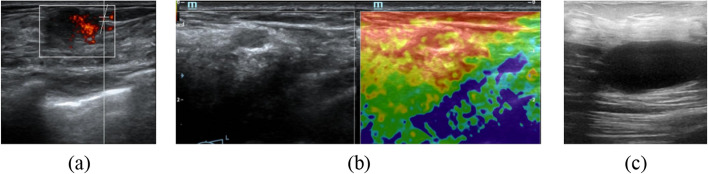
Representative ultrasound image with a the arterial blood flow signal, b B-mode sonography on the left and elastography on the right in the local dataset, and c B-mode sonography in the public dataset_BUSI
